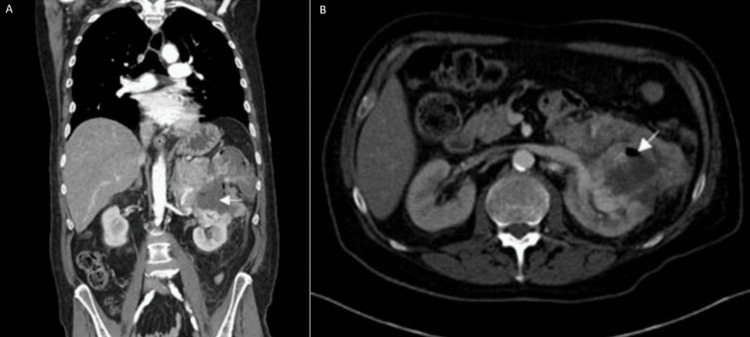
Radiographic images of a 69-year-old male with a history of renal cell carcinoma presenting acutely with back pain and rigors. Abdominal CT scan in A) coronal view and B) axial view. Arrows point to the area of the necrotic renal mass invading the colon.
